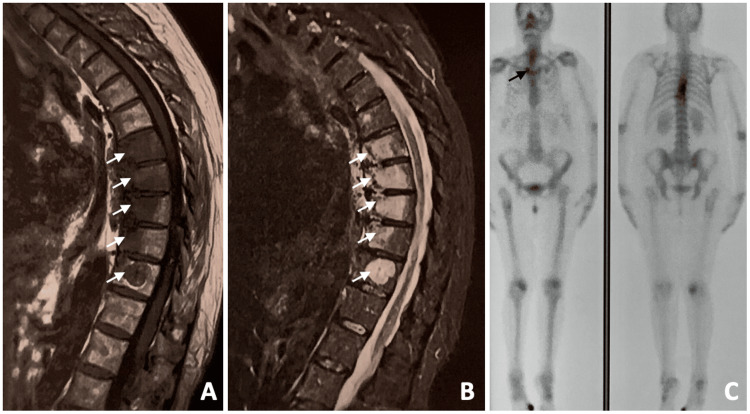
Sagittal T1-weighted (A) and T2-weighted (B) MRI showing signal abnormalities in multiple thoracic vertebral bodies (white arrows). Whole-body bone scintigraphy showing increased tracer uptake in the sternum, right sternoclavicular joint (black arrow), and thoracic spine (C).
